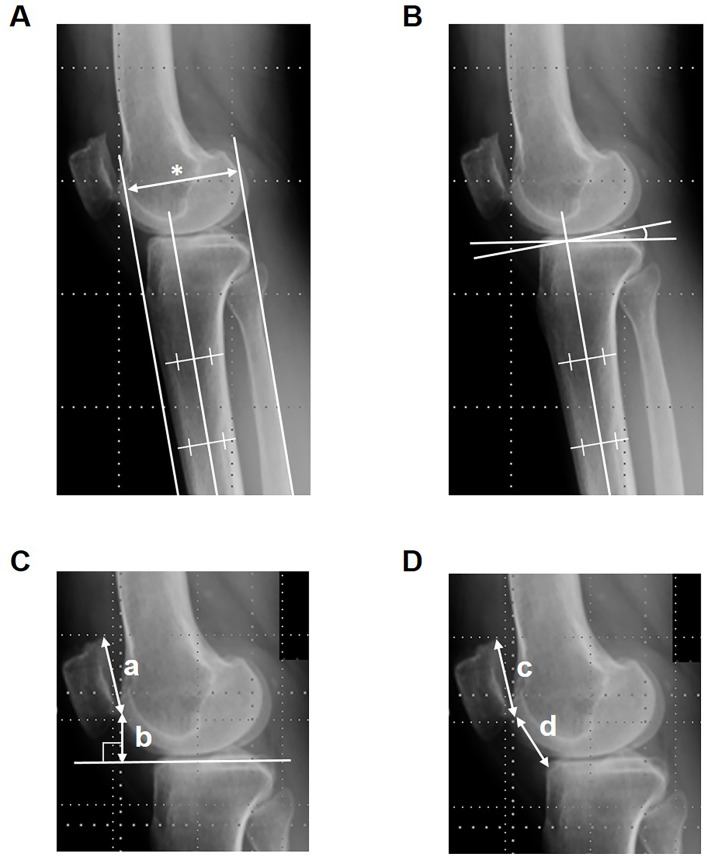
Radiographic measurements. (A) Distance between the anterior border of the tibial tuberosity and the posterior border of the medial tibial condyle (*). (B) Posterior tibial slope. (C) Modified Blackburne-Peel index (b/a). (D) Modified Caton-Deschamps index (d/c).
